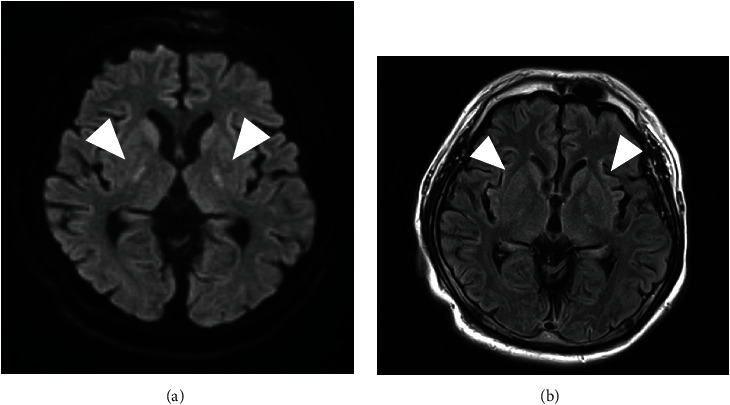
MRI on day 10 revealed only symmetrical hyperintensity in the putamen and posterior limb of the internal capsule (arrow). (a) DWI; (b) FLAIR. DWI: diffusion-weighted image; FLAIR: fluid-attenuated inversion recovery; MRI: magnetic resonance imaging.
